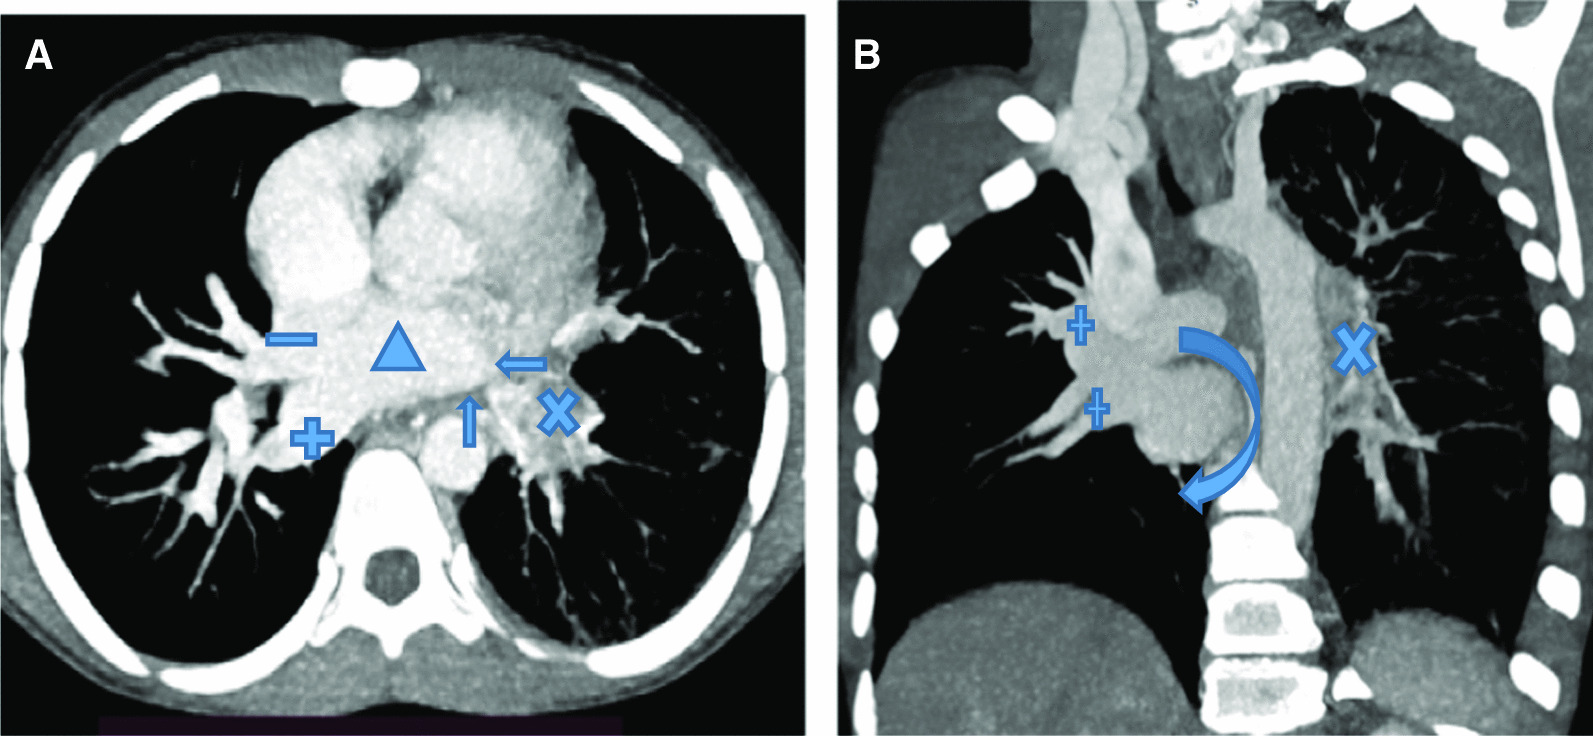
A Axial view Computed tomography angiography showing Left Atrium (∆), right upper (−) and right lower ( +) pulmonary veins. Left boarder of left atrium is smooth (arrows). Vascular anastomosis appears as a left hilar ill-defined mass (x). B Left posterior corona image of Computed tomography angiography with smooth Left Atrium (curved arrow) on the left and branching pulmonary veins on the right ( +). Left hilar vascular anastomosis (x) also seen
